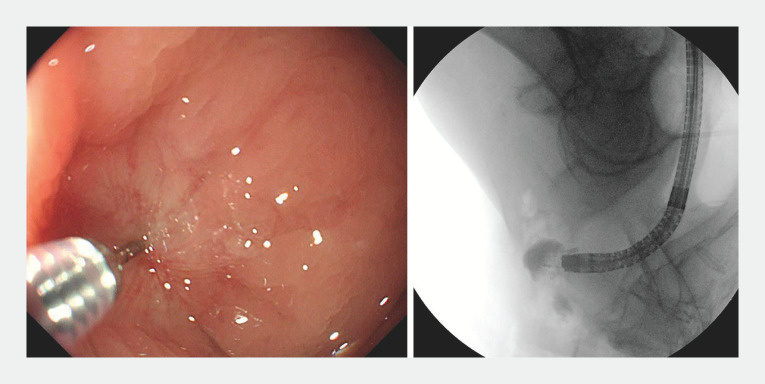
Injection needle puncture in the center of the atresia. X-ray fluoroscopy revealed the appropriate localization of the contrast medium within the distal intestinal lumen.
